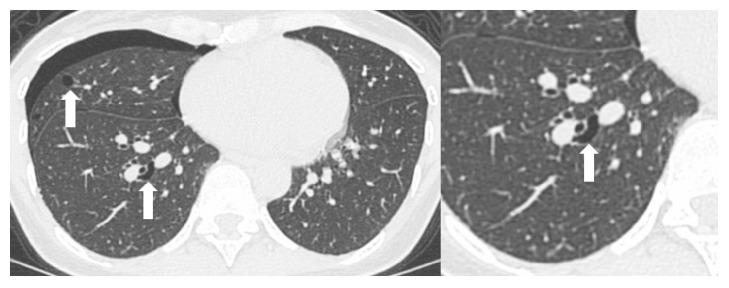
Pulmonary computed tomography demonstrating a right pneumothorax and multiple cysts (arrow). An ellipsoidal cyst abuts on the proximal portion of the pulmonary artery.
